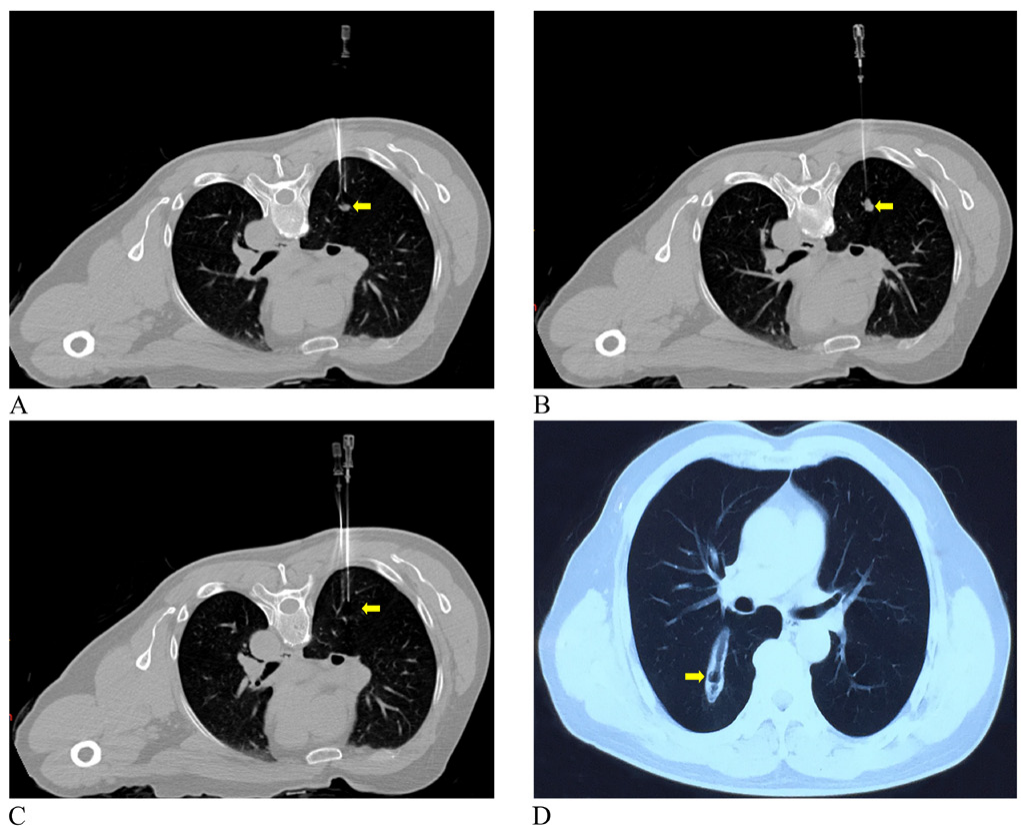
A 64-year-old man with lung metastasis from sigmoid colon cancer Coronal unenhanced CT images revealed laser fibers ablating the tumor 1 cm outside the lesion (A. Before ablation; B. After ablation) (yellow arrows). C. CT image during the two-month follow-up demonstrated completed response (yellow arrow).
